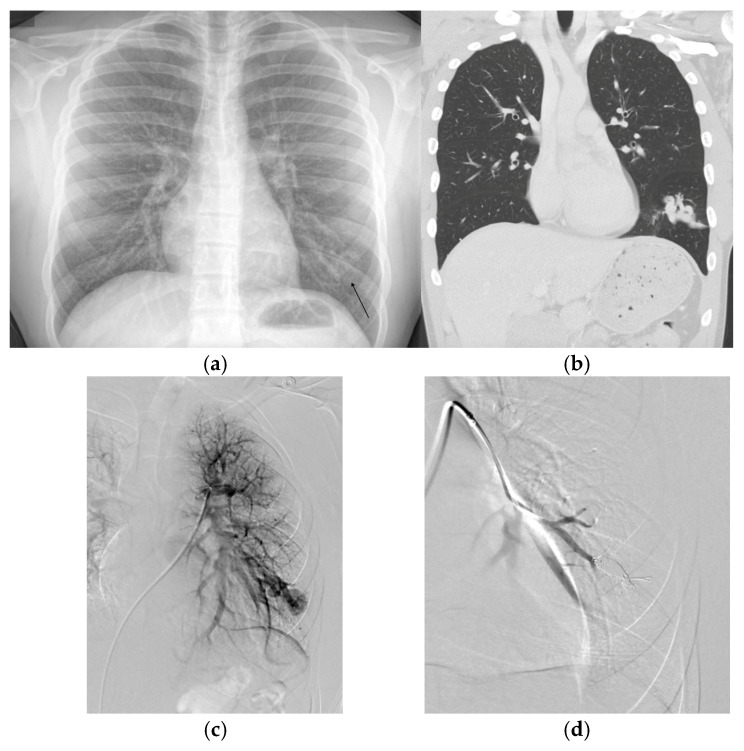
(a) Chest radiograph performed on an adolescent patient for shortness of breath and exercise intolerance demonstrating an opacity in the left lower lobe found to be a large pulmonary arteriovenous malformation (PAVM). The patient was subsequently diagnosed with hereditary hemorrhagic telangiectasia. (b) A subsequent chest computed tomography (CT) demonstrated the complex left lower lobe (LLL) PAVM. A non-contrast coronal image is displayed. (c) The corresponding pulmonary angiogram demonstrating the complex LLL PAVM. (d) Post embolization angiogram demonstrating successful occlusion.
